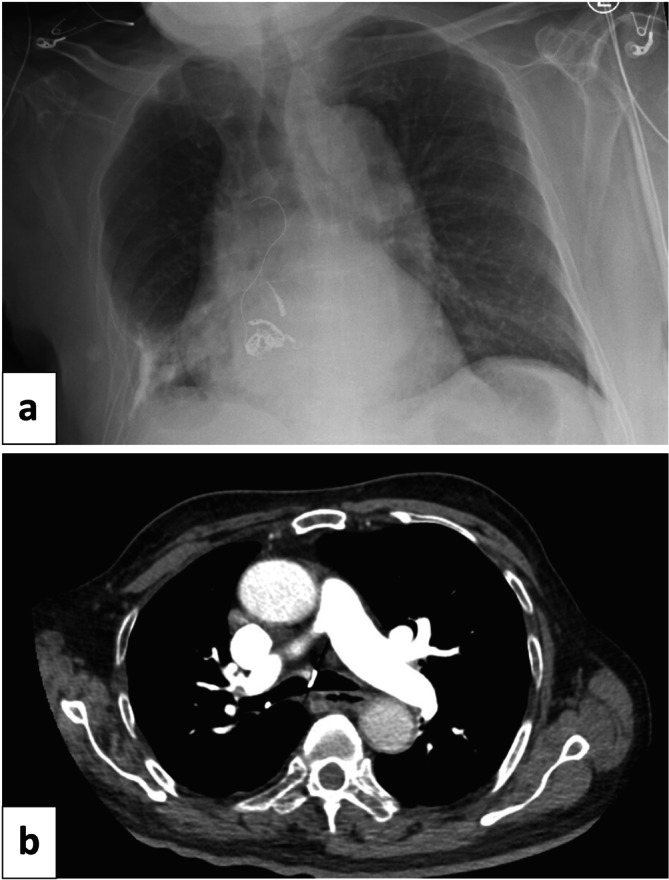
(a) Chest x‐ray demonstrated partial unwinding of one of the embolization coils. (b) Chest CT confirmed endobronchial coil migration to the level of the carina.
